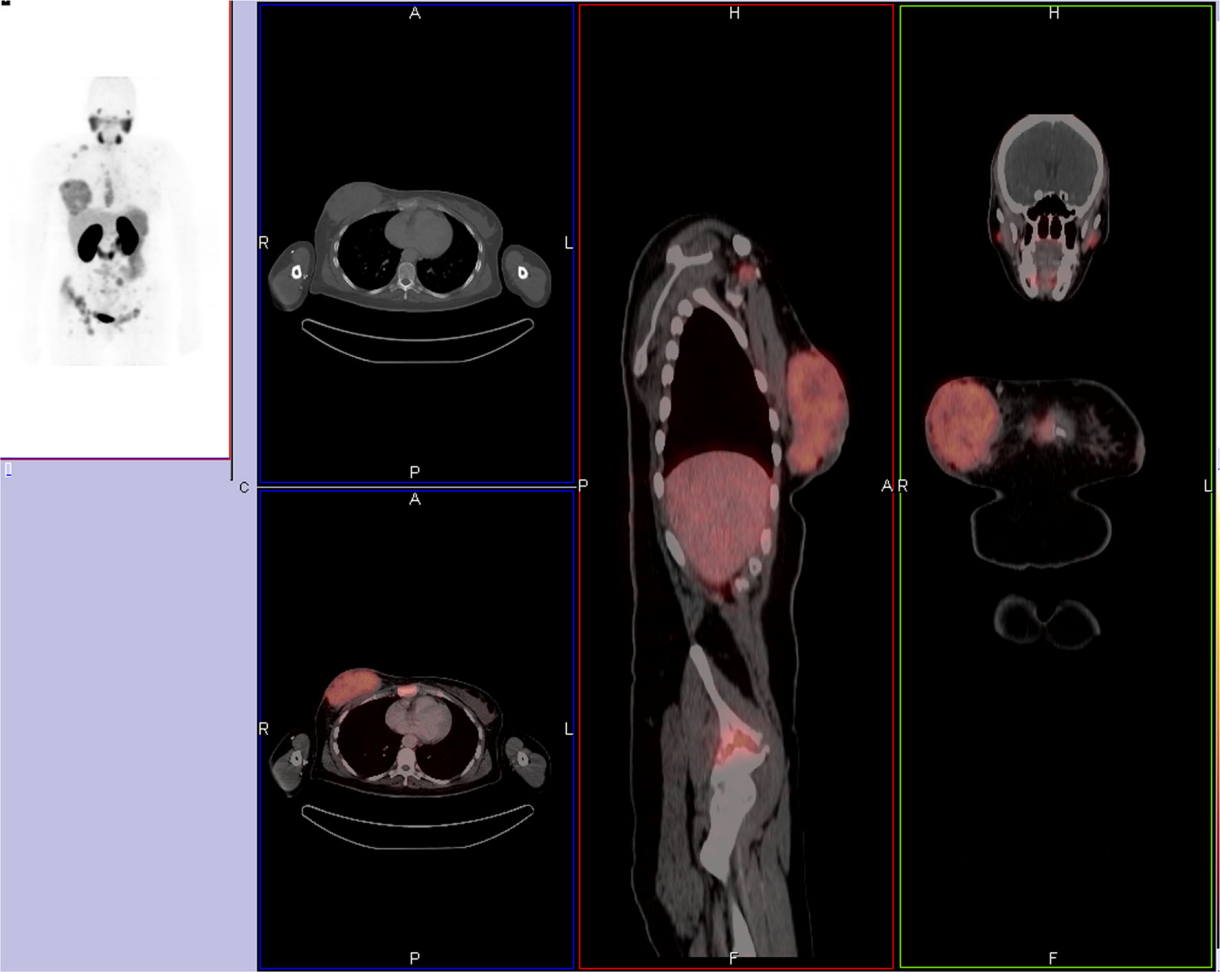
A 39-year-old woman with stage IV by ^68^Ga-PSMA PET/CT. a Maximum-intensity-projection PET demonstrated multiple osseous metastasis and a primary right breast cancer. Axial, coronal, and sagittal fused PET/CT confirms all the lesions (b)
